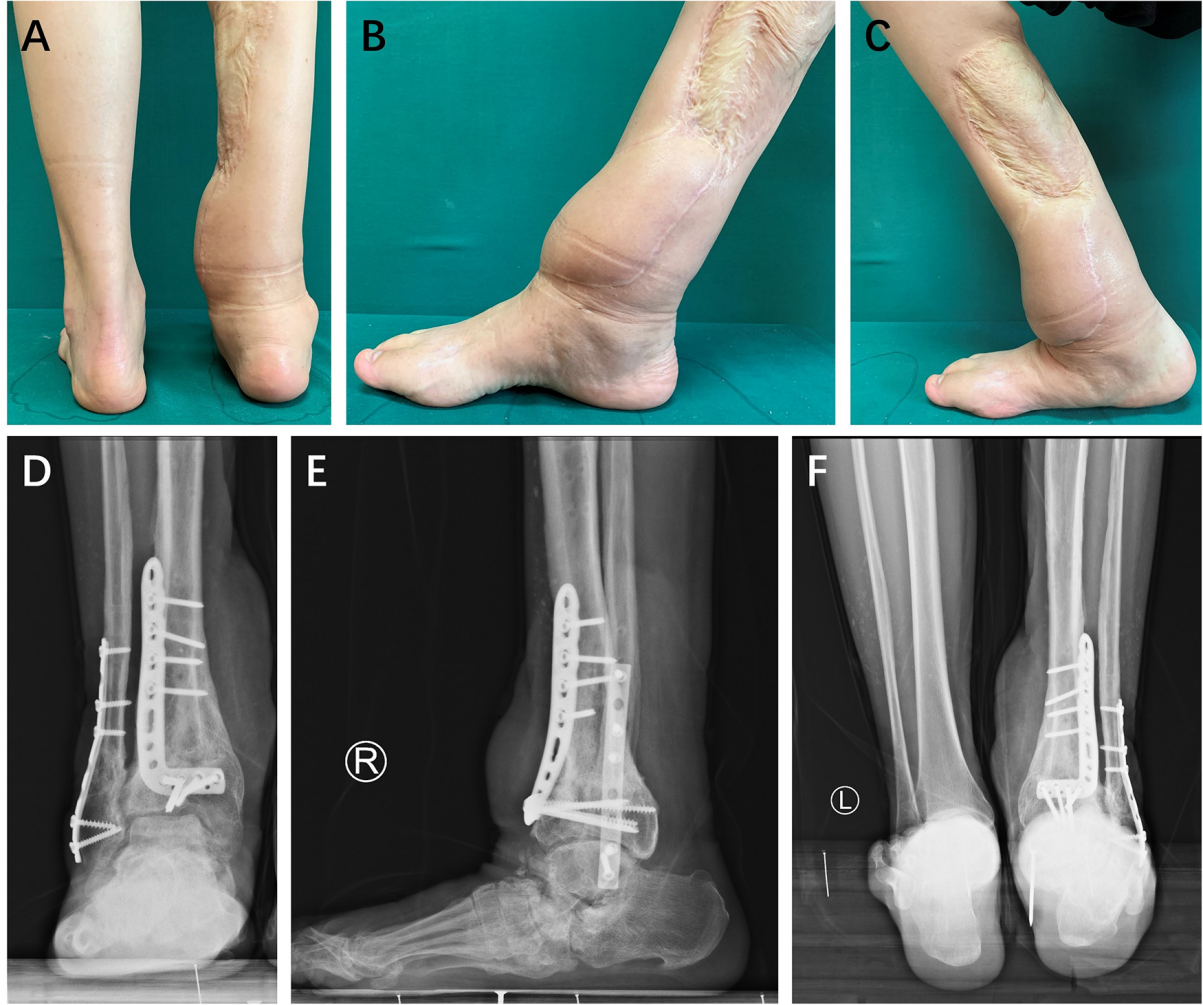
Three years after the supramalleolar osteotomy, the patient showed satisfactory hindfoot alignment (A) and an adequate range of motion (B,C). Radiographic images revealed the presence of osteophytes anterior to the talus (D,E). The hindfoot alignment remained satisfactory (F).
